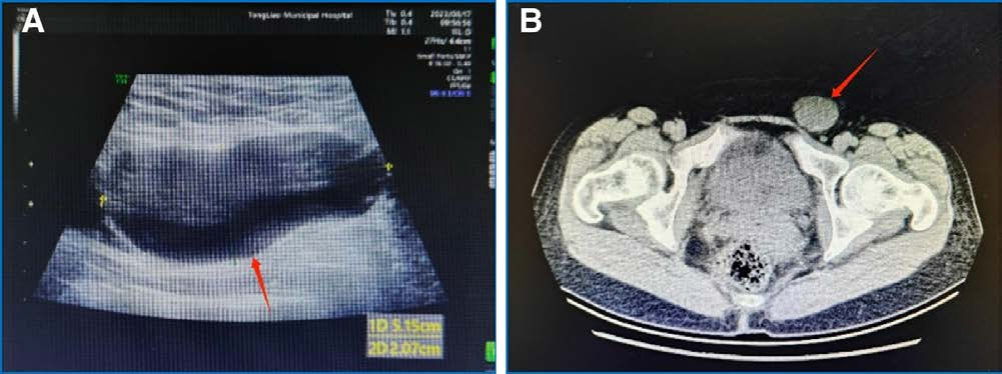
(A) Ultrasonography showed a cystic mass, smooth cyst wall, low echo signal, and no detectable blood flow signal (red arrow). (B) CT showed a clear, regular, low-density cystic mass in the left groin area (red arrow). CT = computed tomography.
